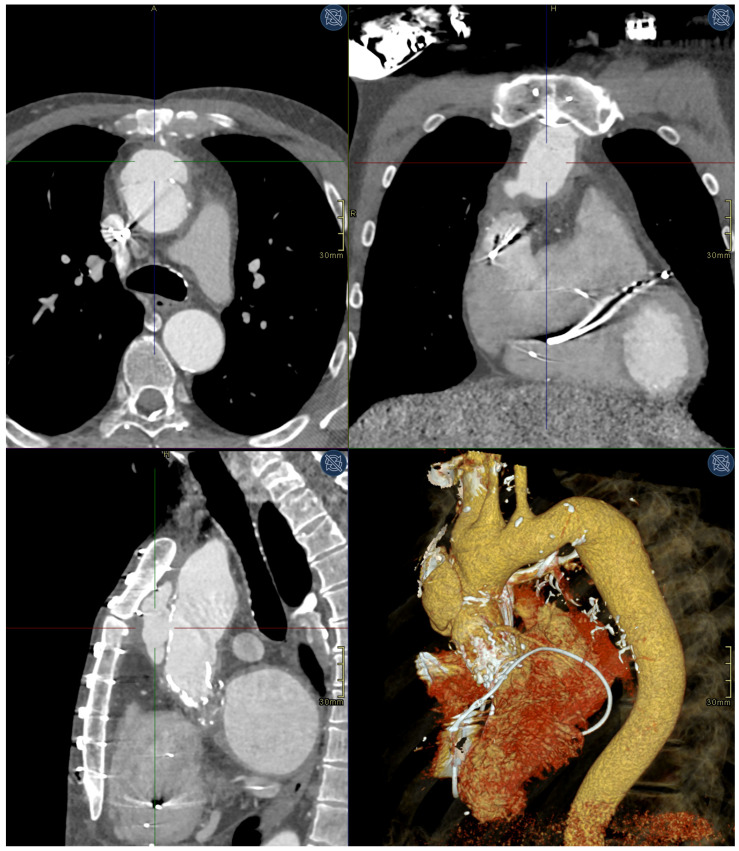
Preprocedural CTA showing a 58 × 55 × 30 mm pseudoaneurysm anteriorly in the ascending aorta. Clockwise from left bottom: sagittal plane arterial phase CTA, axial plane arterial phase CTA, coronal plane arterial phase CTA, volume rendering technique (VRT) 3D representation CTA.
